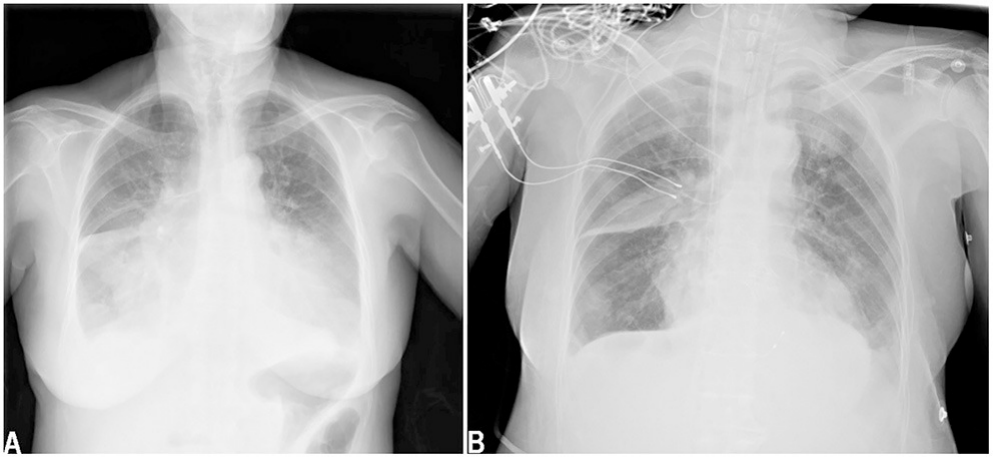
Chest x-ray showed pulmonary oedema and bilateral pleural effusion when admission (A). Chest x-ray showed pulmonary oedema and bilateral pleural effusion decreased after TAVR (B).
